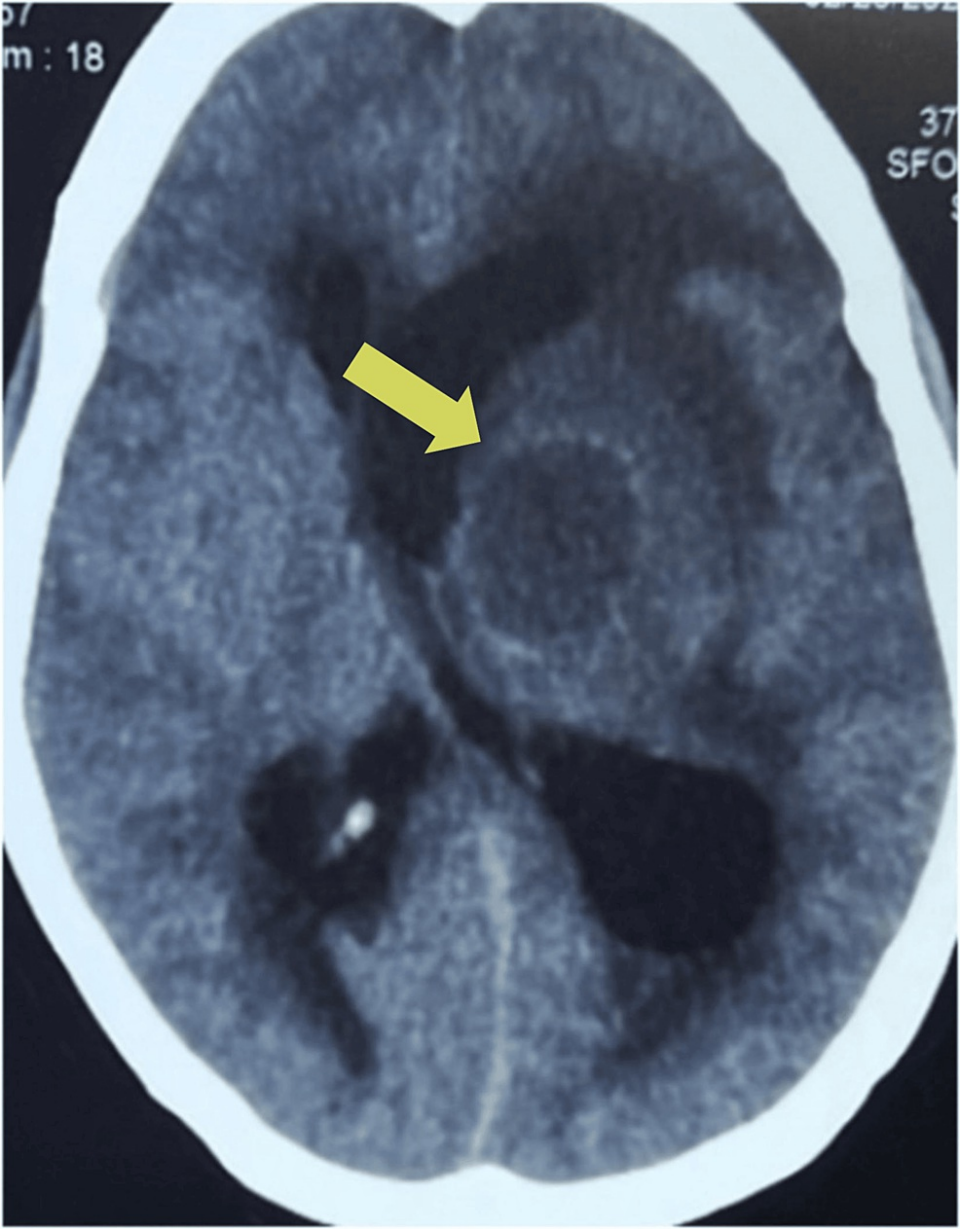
NCCT brain showing a 23.8×23.5×27.6 mm well-defined hypodense lesion in the left perisylvian region and thalamus with surrounding cerebral edema causing midline shift toward the right with contralateral compression of the right lateral ventricle. NCCT, non-contrast-enhanced computed tomography
